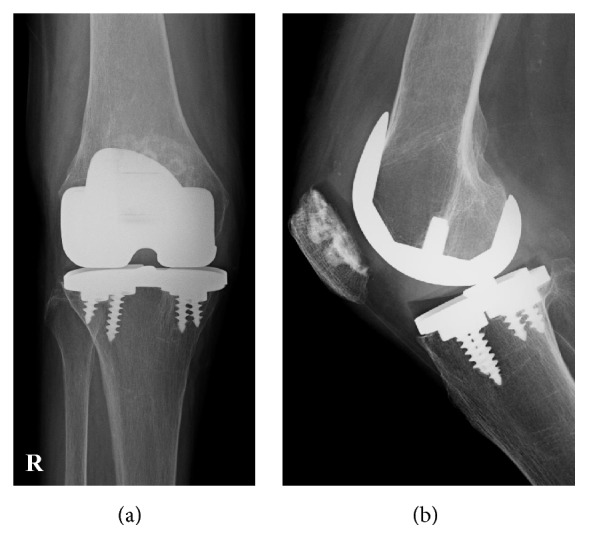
Follow-up plain radiograph obtained 17 years after initial surgery of the right-side implants shows normal alignment is maintained, with no evidence of stress shielding or loosening.
